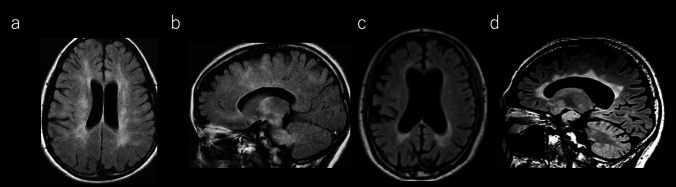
Representative case of leukoencephalopathy-like lesions. Brain magnetic resonance images from case 34 at two time points. Axial Fluid-attenuated inversion recovery images at onset (a, b) and 15 years after symptom onset (c, d) show the evolution of white matter lesions. Initially, the lesions were ill-defined and bilaterally distributed (a, b). At follow-up (c, d), the lesion boundaries became well-demarcated and vertically oriented along the lateral ventricles, consistent with Dawson’s fingers. This case demonstrates radiological conversion to a typical multiple sclerosis pattern over time
